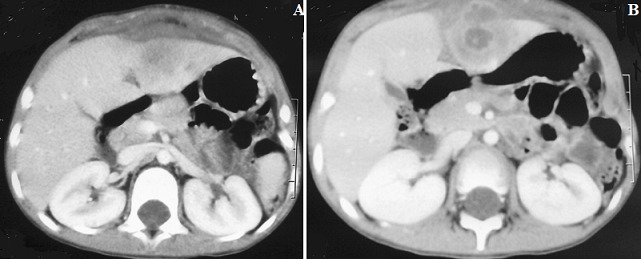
A) CT scan showing abscess involving in the left lobe of liver with extension into the overlying anterior abdominal wall; B) the early phase of the contrast-enhanced CT scan revealed a strongly enhanced margin of the mass liver and no enhancement of the inside of the mass
